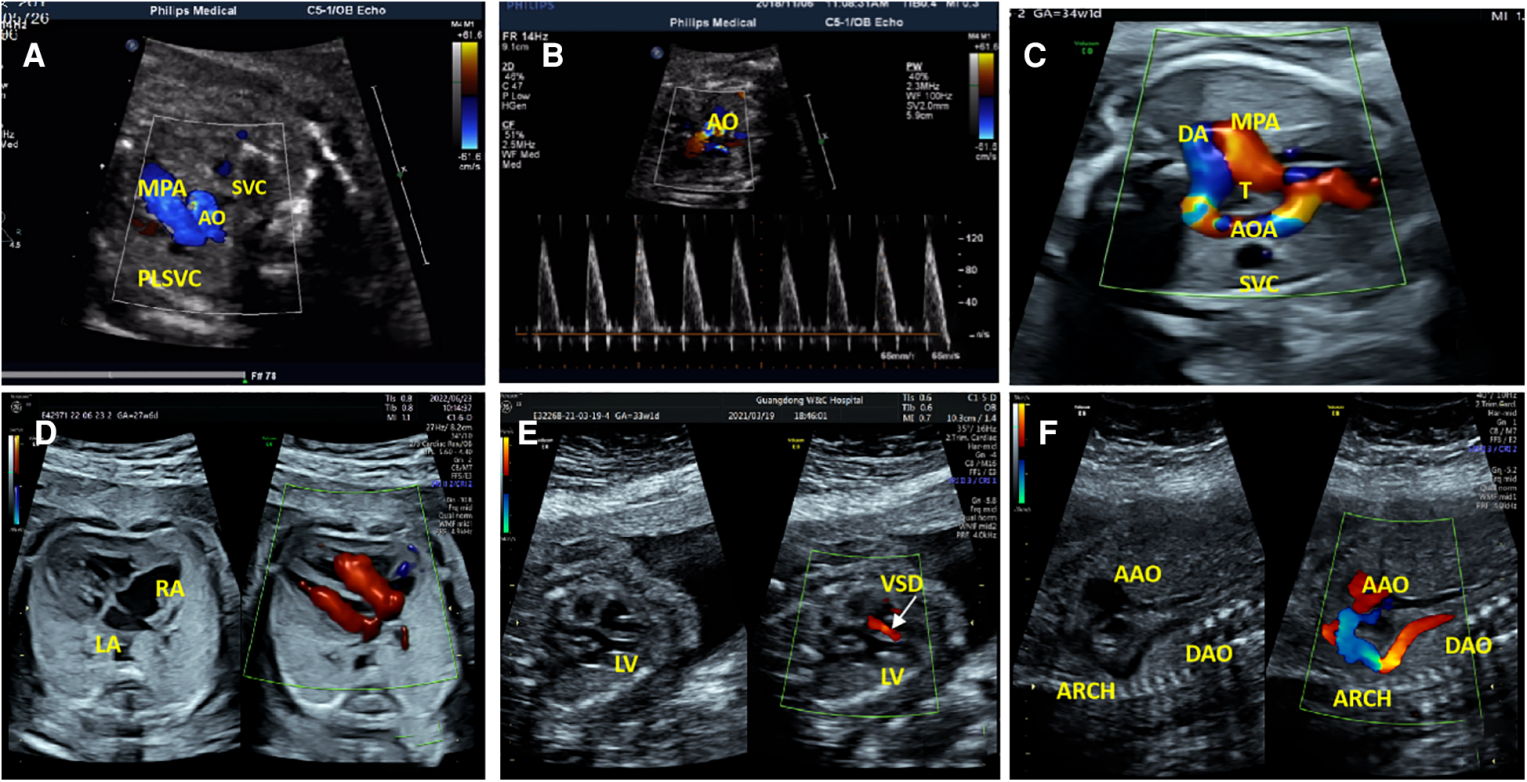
The most common cardiovascular abnormalities in our study. (A) The ultrasound examination of case 1 showed a small additional vessel found at the main pulmonary artery (MPA) left in the three-vessel view. SVC, Superior Vena Cava; AO, Aorta; PLSVC, Persistent Left Superior Vena Cava. (B)The color Doppler ultrasonic images of case 1 in the left ventricular outflow tract view showed elevated blood flow rate of the aortic valve. (C)The ultrasonic examination of case 7 showed that the aortic arch was on the right side of the trachea (T), the MPA was on the left side of the T, and they constituted U-shaped ring of blood vessels at the three-vessel view. AOA, Aortic Arch; DA, Ductus Arteriosus. (D) Two-dimensional and color Doppler ultrasonic images of case 9 showed enlargement of the right atrium (RA) in the four-chamber view. (E)Two-dimensional and color Doppler ultrasonic images of case 14 showed left ventricular septal defect (VSD), and left-to-right shunt of defect part (color Doppler flow signal at the defect) in the left ventricular outflow tract view. LV, Left Ventricle. (F) Two-dimensional and color Doppler ultrasonic images of case 14 showed that the descending aortic arch extends to the left obviously in the long axis of the aortic arch view. AAO, Ascending Aorta; DAO, Descending Aorta.
